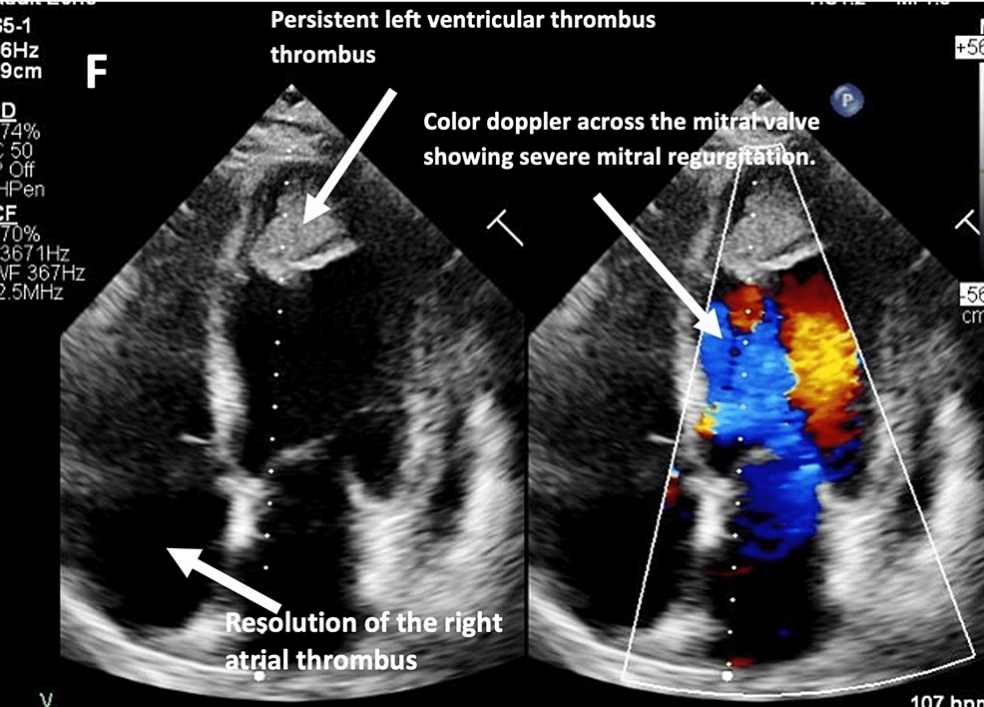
Transthoracic echocardiogram apical four-chamber view with color Doppler in the right picture showing interval resolution of the RA thrombus, with no change in the LV thrombus size along with severe mitral regurgitation (arrows). LV: left ventricle, RA: right atrium
